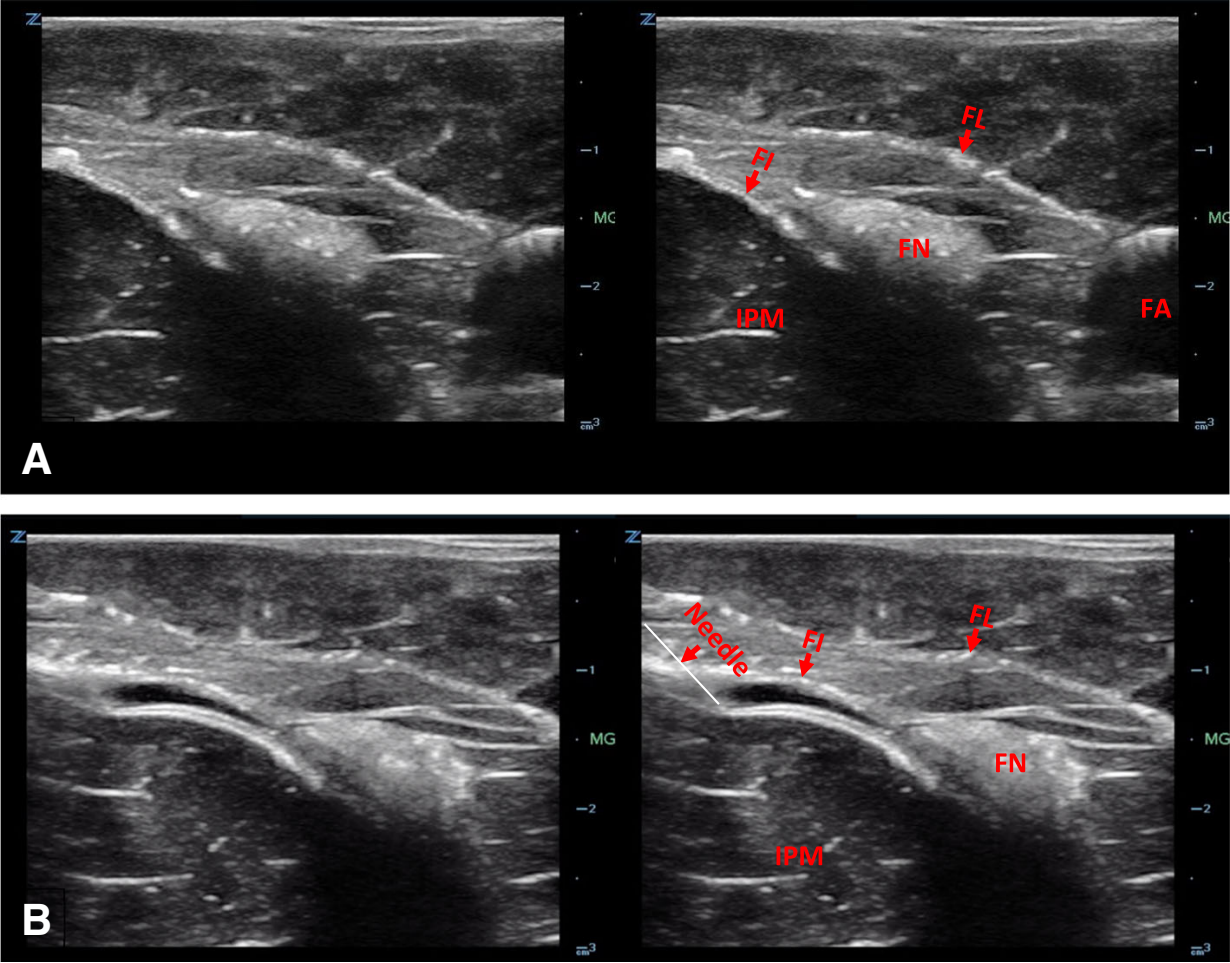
Ultrasound image of the fascia iliaca compartment model (a). Ultrasound image of the needle with hydrodissection of fascia iliaca plane (b). FN, femoral nerve; FA, femoral artery; IPM, iliopsoas muscle; FI, fascia iliaca; FL fascia lata
